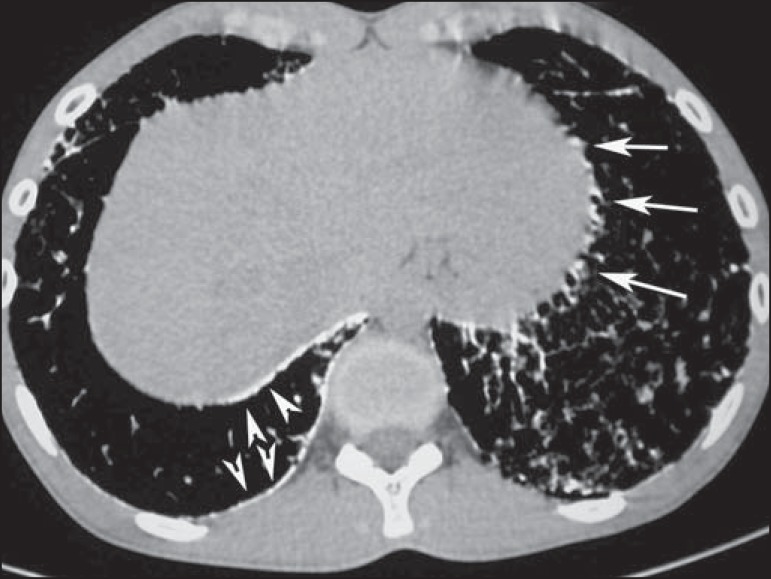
CT with mediastinal window demonstrating small calcified nodules with subpleural
